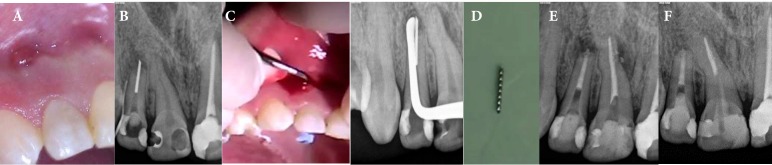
A) Intraoral sinus tract; B) Periapical radiography of the right central and lateral incisors; C) A long shank excavator was passed from the sinus tract to the root tip of the tooth and the broken file was pushed into the canal; D) Retrieved fragment; E) Canal obturated with MTA; F) 18 months after treatment
